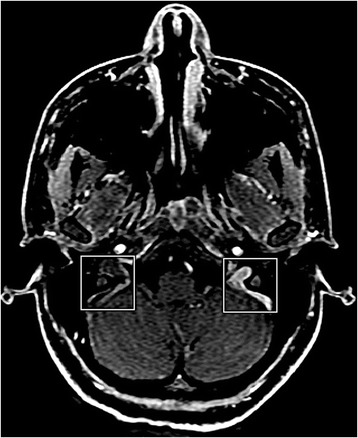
Brain magnetic resonance imaging study showing cerebral venous sinus with signs of thrombosis on right (*squares*)
